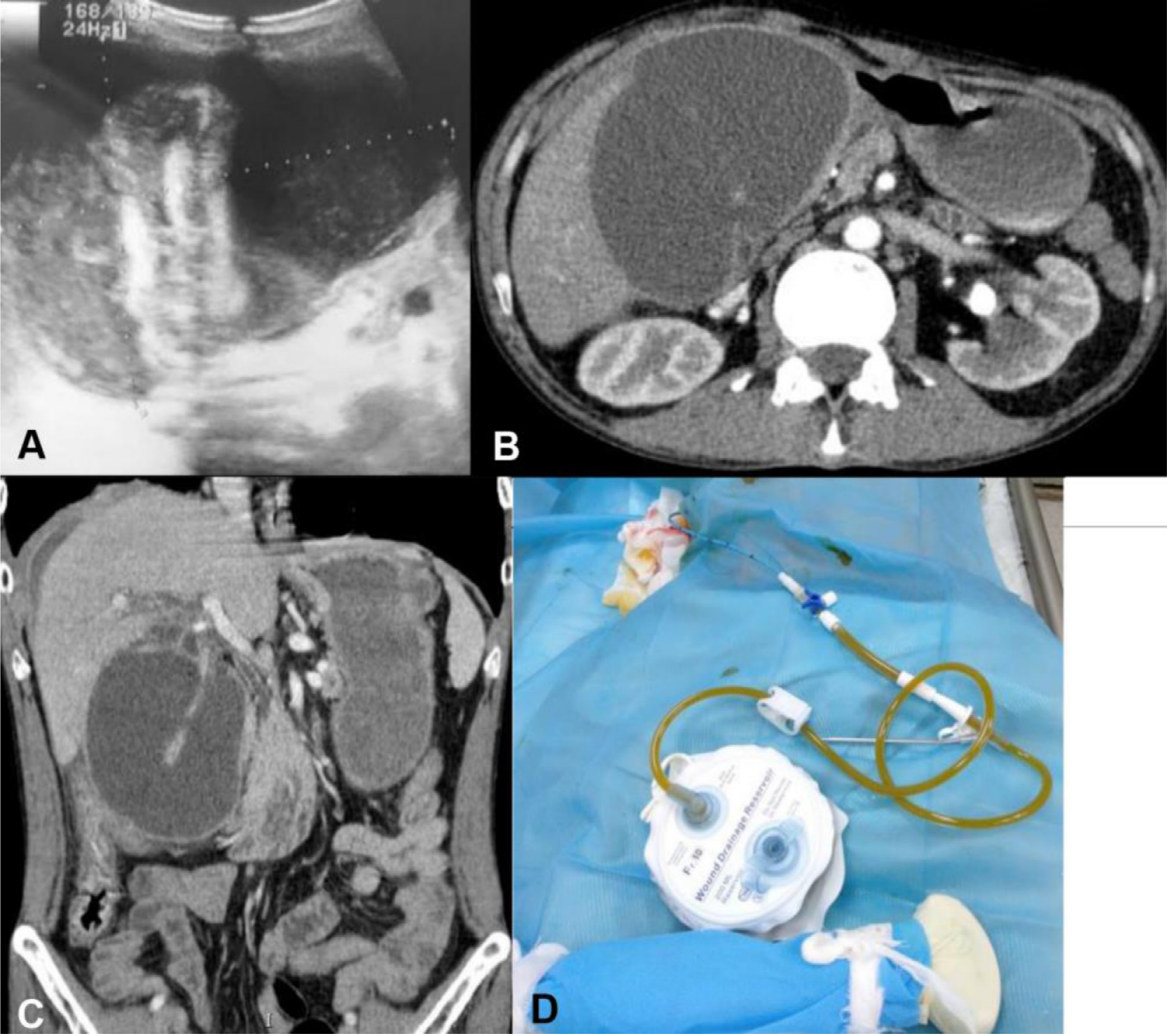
Ultrasound (US) performed on day 17 demonstrated a diffuse gallbladder wall thickness surrounded by a voluminous mass of pericholecystic fluid measuring 9 × 10 × 12 cm (A). Abdominal contrast-enhanced computed tomography (CT) confirmed ultrasound (US) findings and enhancement of a thickened gallbladder wall, free fluid around the liver and the right paracolic gutter was seen (B and C). US-guided percutaneous transhepatic drainage of the gallbladder was performed and around 350 ml of bile fluid was aspirated (D).
